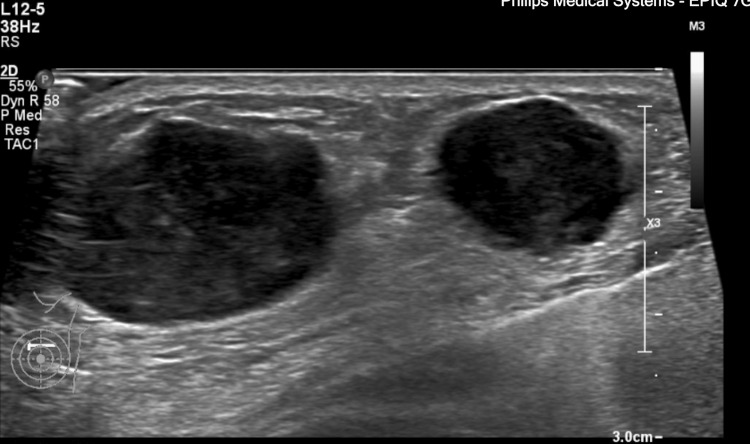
Ultrasound of the left breast with three oval hypoechoic parallel masses, with the largest measuring 2.9 x 1.4 x 2.5 cm.
